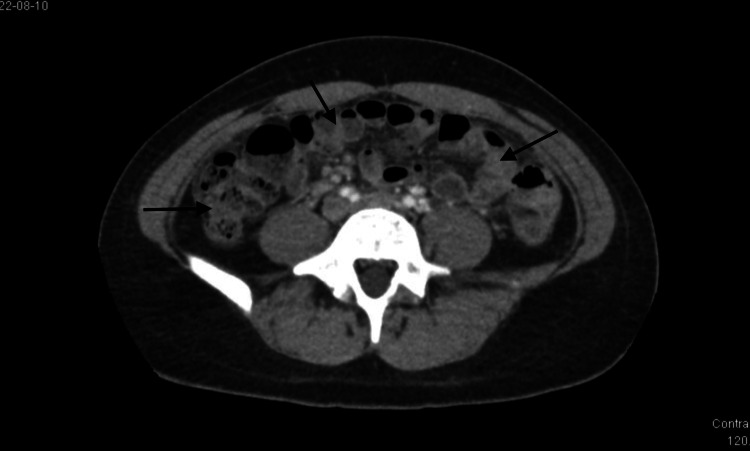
CT abdomen/pelvis (axial plane) The image is demonstrating evidence of intestinal inflammation (arrows) concerning enteritis.
